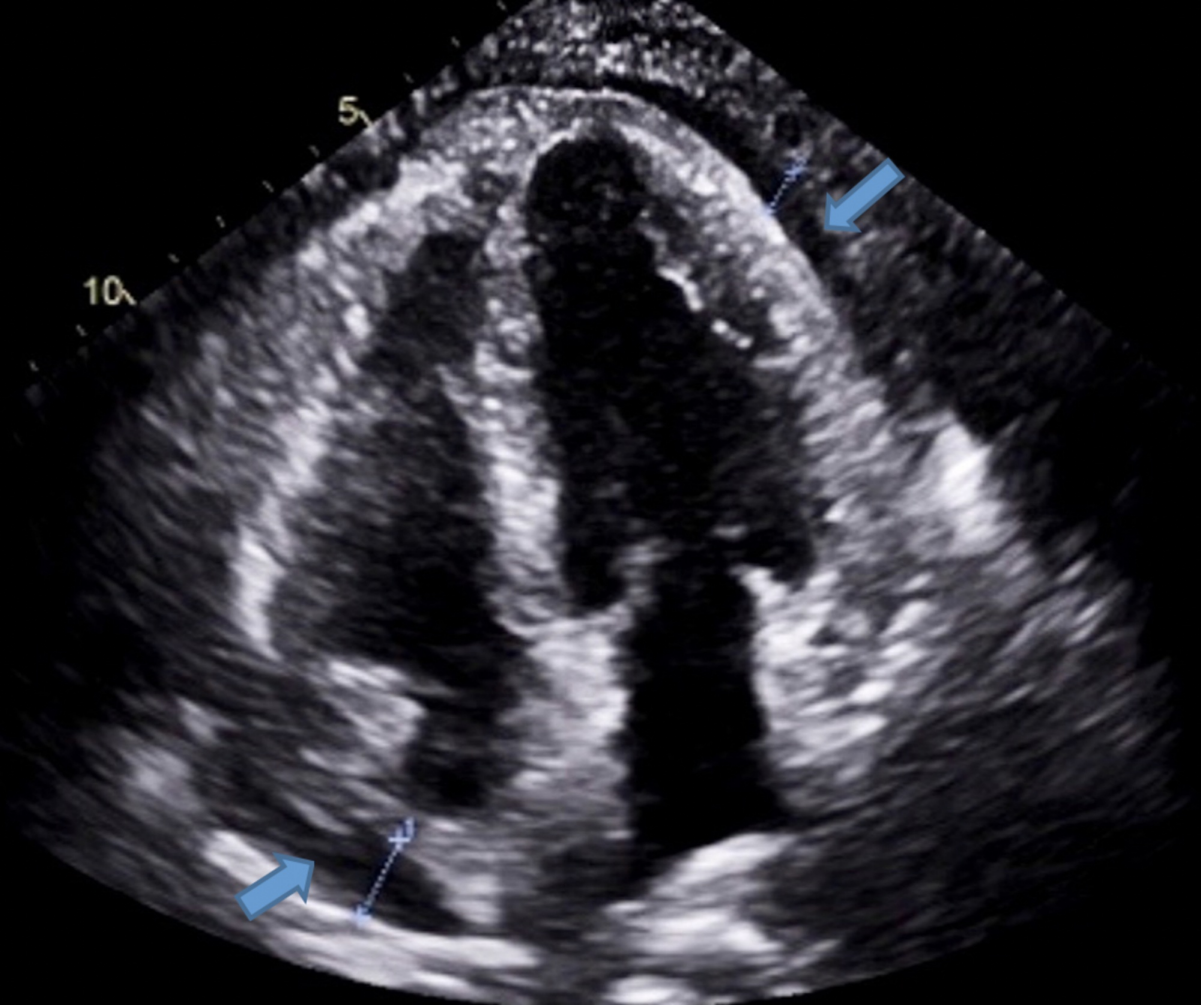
Apical 4 chamber view showing a moderate pericardial effusion.
